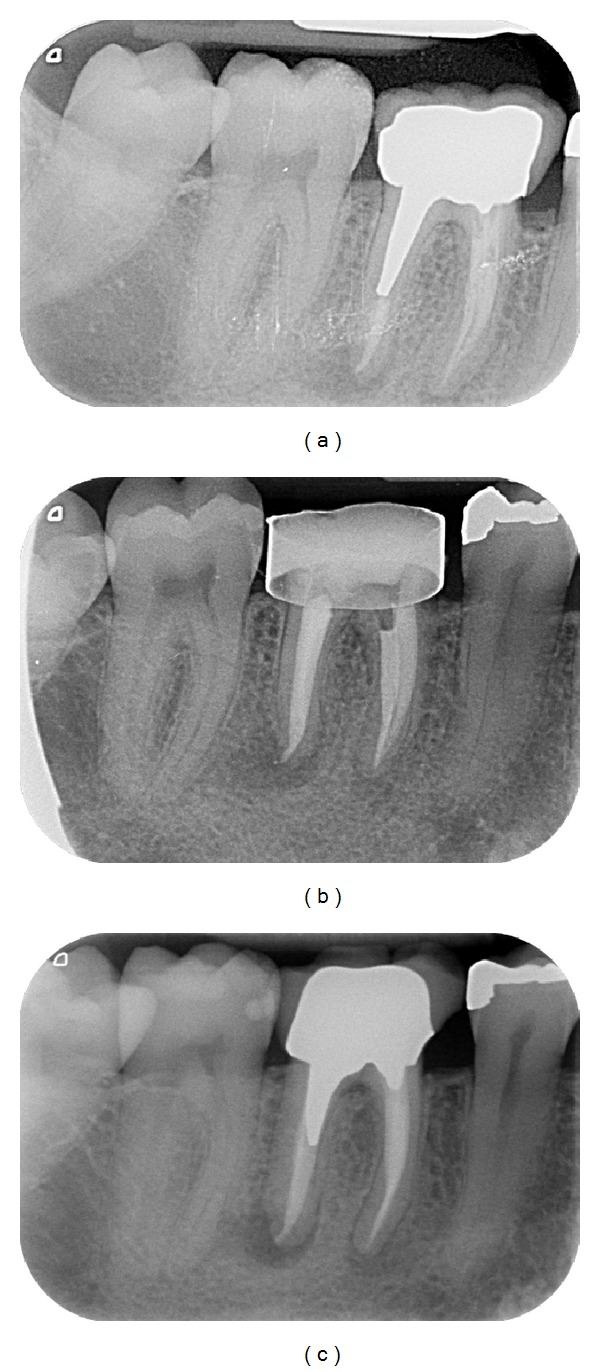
Case classified as failure. (a) Preoperative X-ray of the 46, painful, with an acute apical abcess. The previous endodontic treatment was performed 24 months ago. Note the apical radiolucency (PAI > 3). (b) Periapical X-ray control immediately after the retreatment. Note the copper ring used as preendodontic reconstitution. (c) Follow-up for 4 years: tooth was classified as “failure”: the apical radiolucency is always present and the tooth is symptomatic at the percussion test. The prosthodontics treatment and the coronal seal are questionable.
